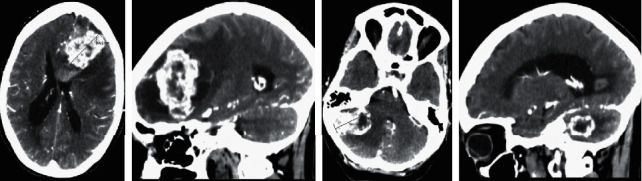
Enhanced CT scan showing 2 lesions with halo of edema, left frontal and right cerebellar. The frontal lesion had an associated bleeding area of 3 cm in diameter.
